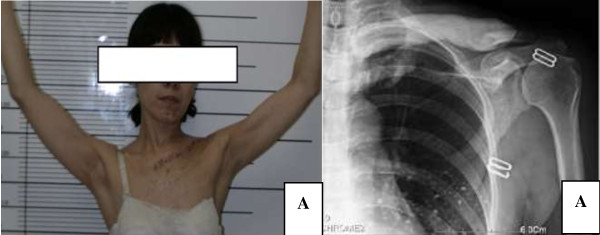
Shoulder outline and X-ray of postoperation. A: Post-operative shoulder outline and functional display. Patient has an aesthetic shape and favorable function B. Radiograph of post bone cement reconstruction shows a fine prosthesis location.
